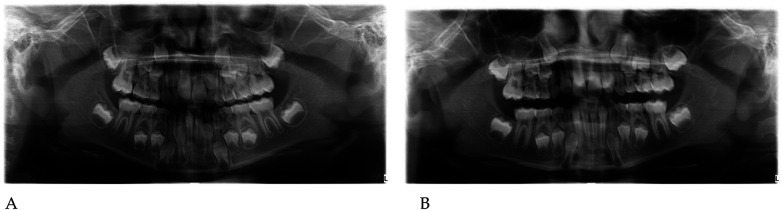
Pre- (A) and post-treatment (B) panoramic radiographs of case 5.
